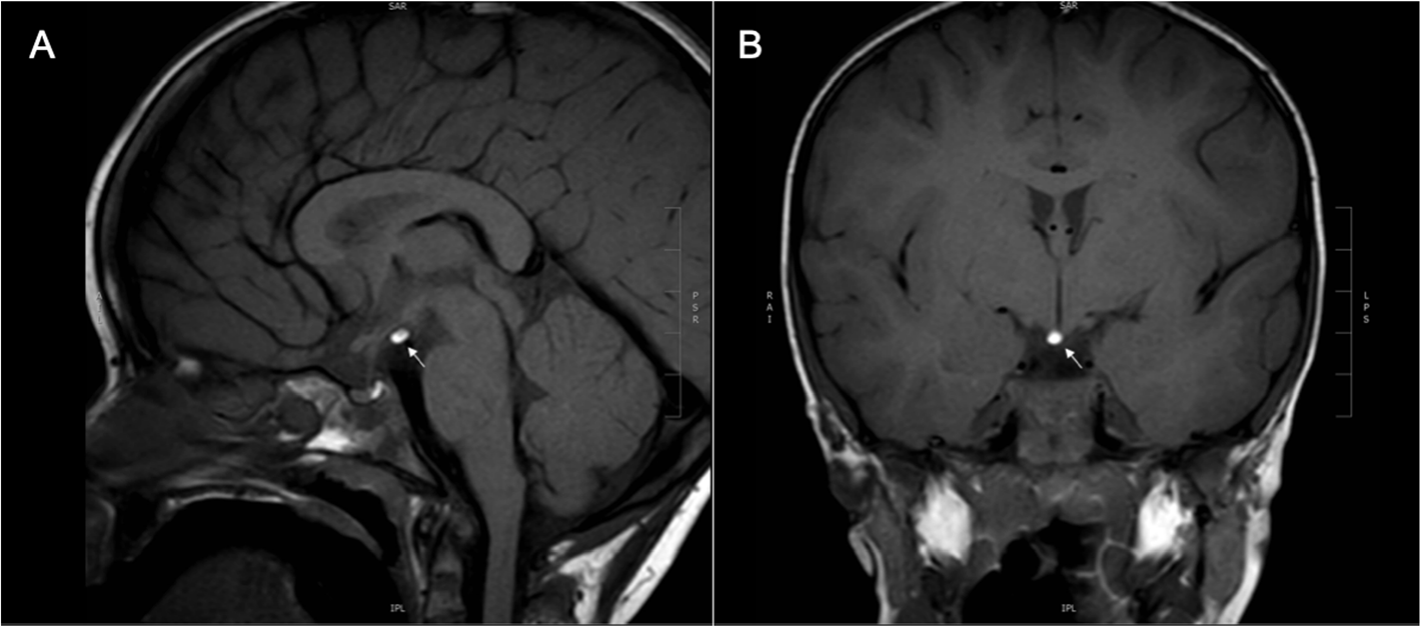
Sagittal (a) and coronal (b) T1-weighted MR images reveal a hypothalamic lipoma. The pituitary gland has a normal signal intensity and no other intra-cranial abnormalities are found
